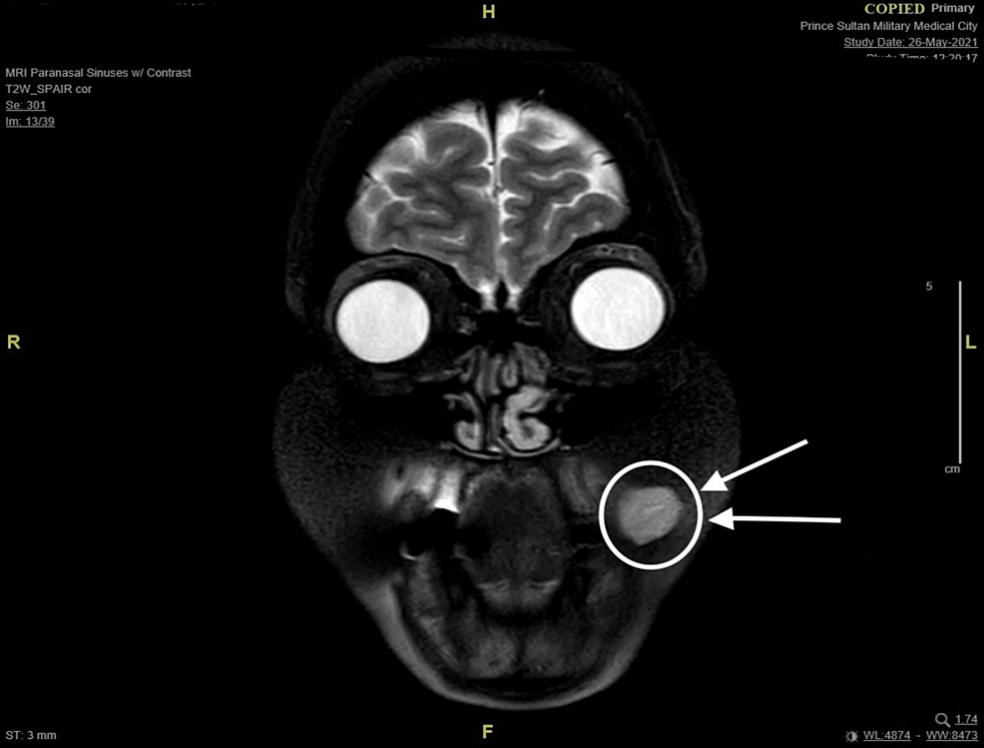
MRI T2-weighted image (coronal cut) demonstrating hyperintense mass post contrast on the left buccal space. MRI: magnetic resonance imaging
